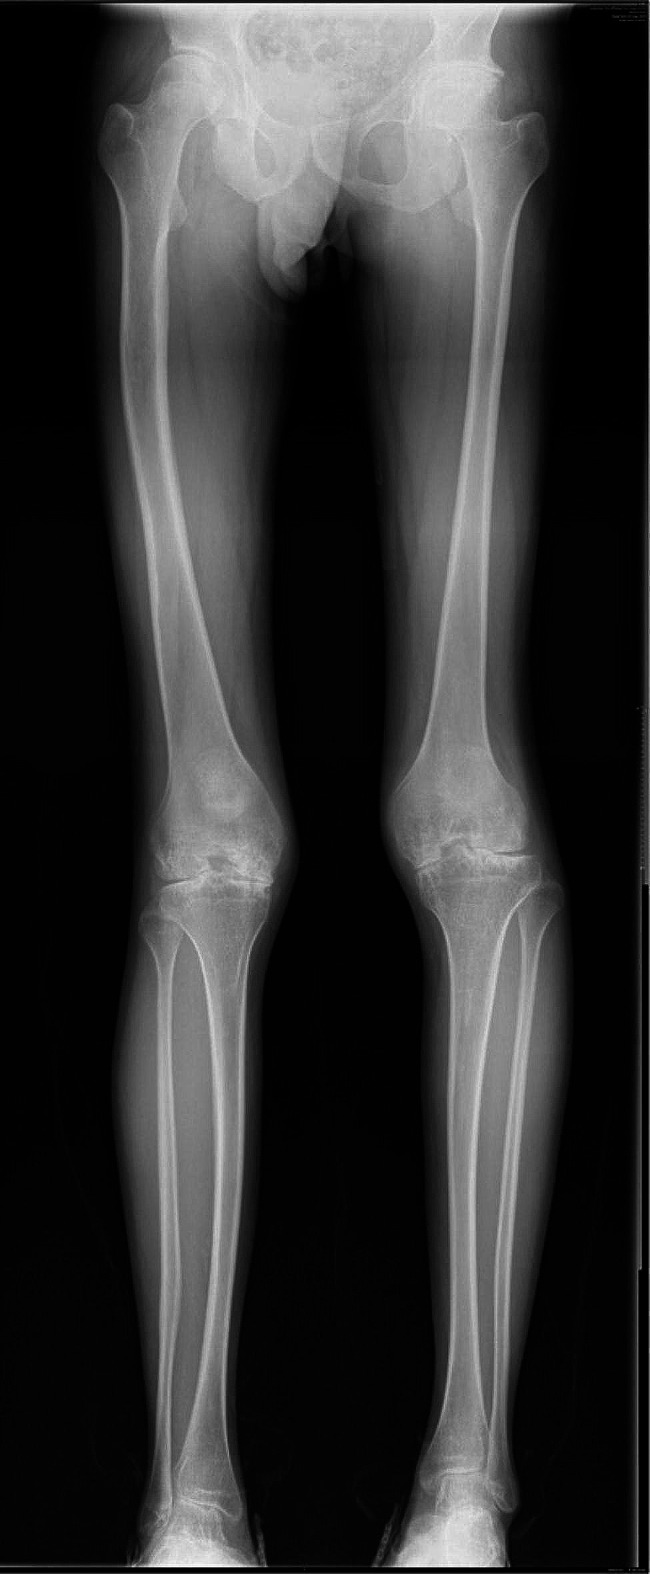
Full-length X-rays of both lower limbs showed a significant narrowing of joint spaces, severe sclerotic changes, and pronounced deformities
